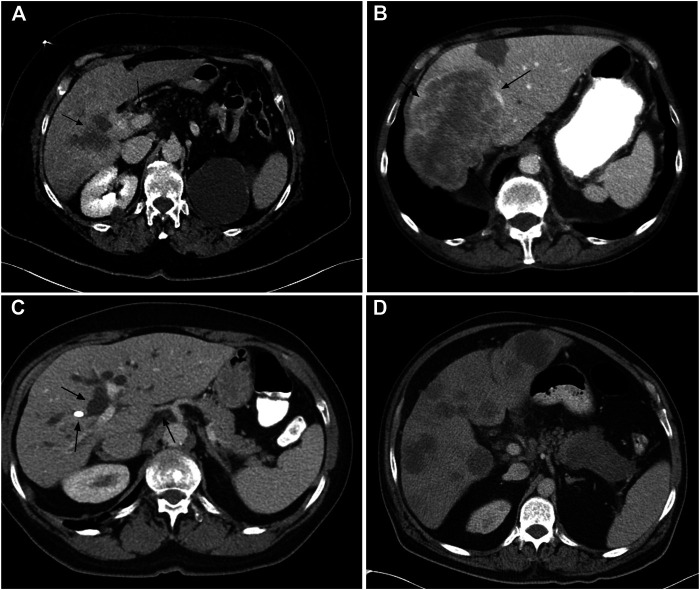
CT scans from the study showing: Mass forming hilar CCA with infiltration along portal vein (A, arrows toward the tumor mass); Large mass forming peripheral CCA (B, arrows toward the tumor mass); Hilar CCA with bile duct dilatation, stent and infiltration along hepatic artery (C, arrows showing the bile duct dilatation); Pancreatic tail tumor with multiple liver metastases (D).
